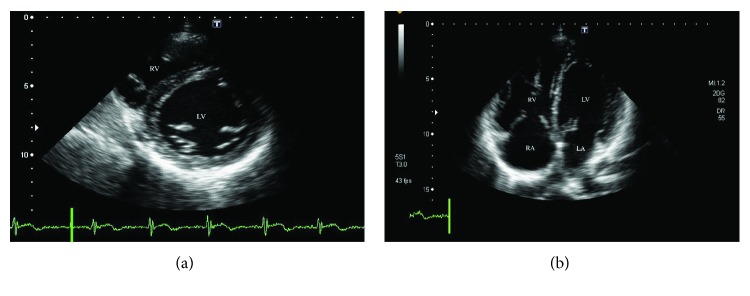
Follow-up echocardiography, 6 months after the upfront combination therapy. (a) Short axis view of the TTE showed no compression of the interventricular septum. (b) Apical four chambers view the TTE showed improvement of the right ventricular size. TTE: transthoracic echocardiography; LV: left ventricular; LA: left atrium; RV: right ventricular; RA: right atrium.
